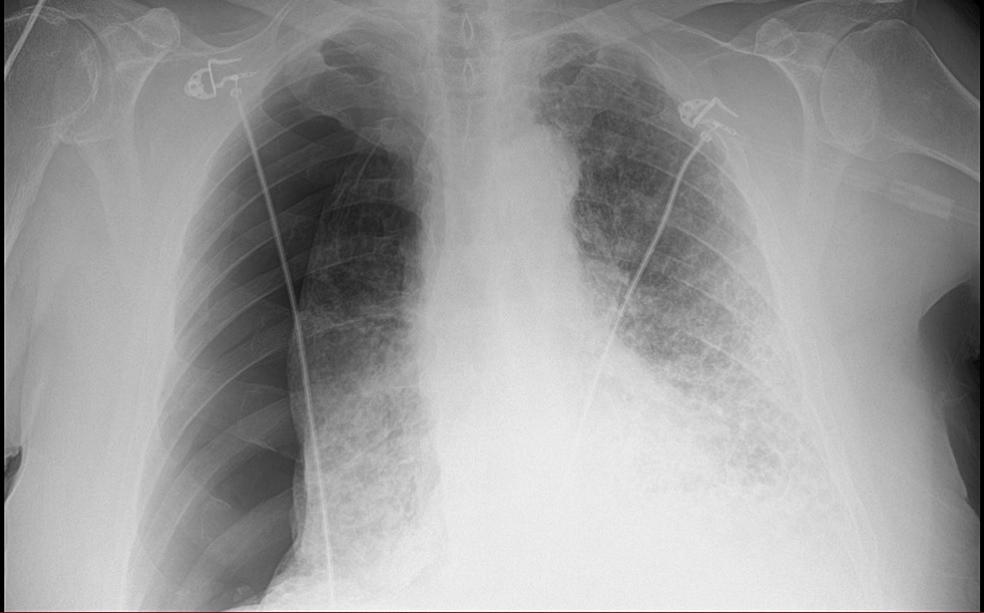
Right-sided pneumothorax with a collapsed lung. Anterior-posterior (AP), portable chest X-ray.
